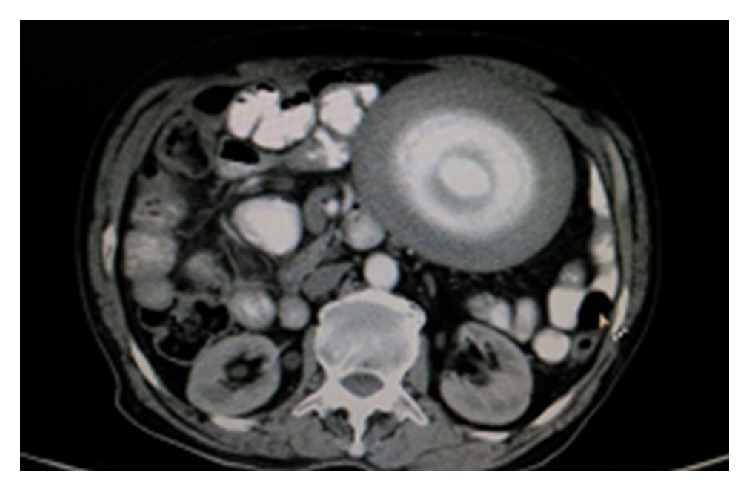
Contrast CT abdomen showing concentrically calcified mass.
